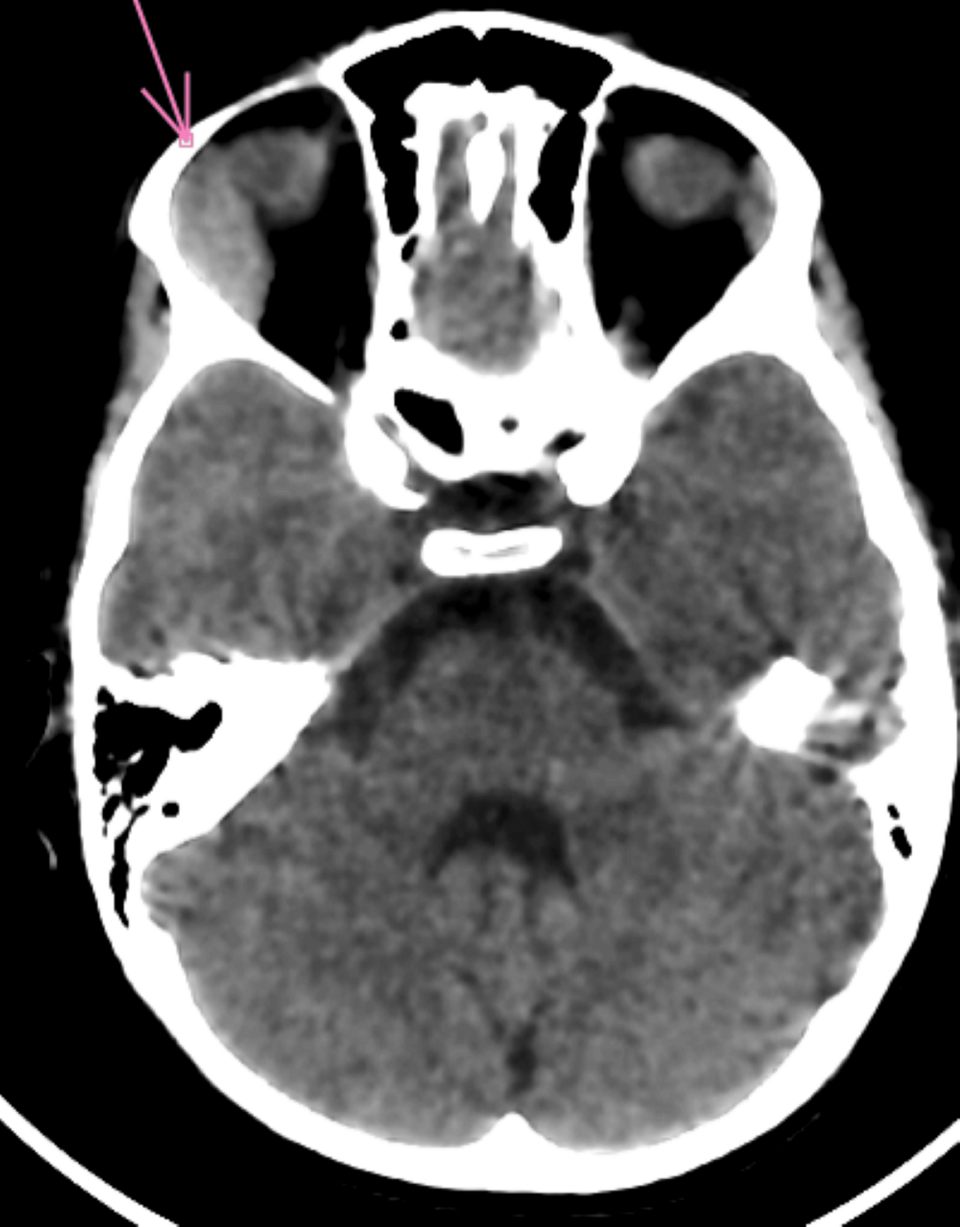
Axial non-contrast brain-orbit CT scan showing infiltration of the lacrimal gland with extraconal extension
